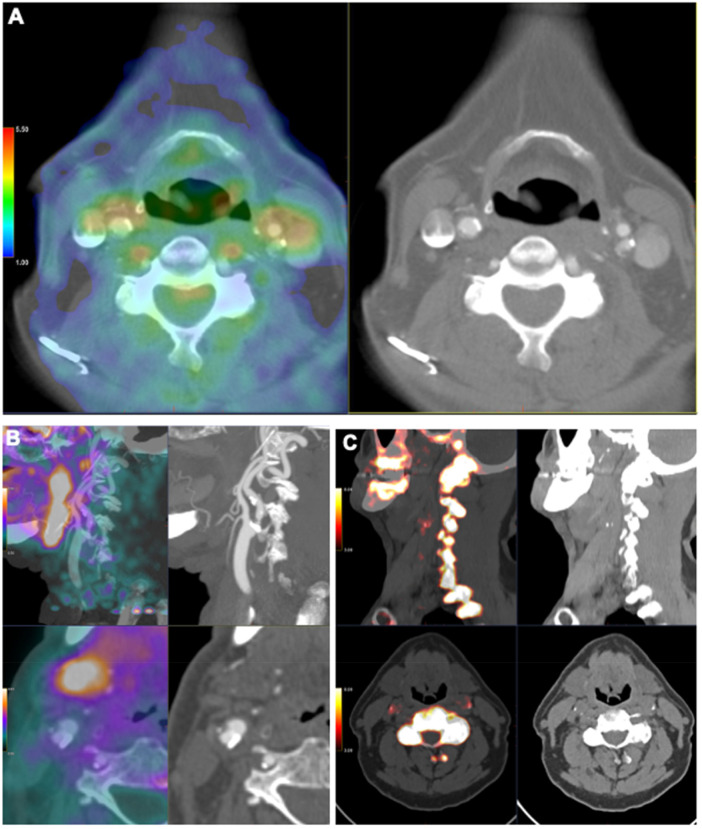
Representative figure demonstrating research examples of PET tracers used to study atherosclerotic plaque. (A): the panel on the left shows used FDG PET/CT angiogram image showing higher activity at the left carotid plaque at the level of proximal internal carotid and on the right the corresponding CT angiogram image showing mixed calcified and non-calcified plaque components; (B): left panels showing fused Ga^68^ DOTATATE PET/CT angiogram image showing higher activity at the right carotid plaque at the level of common carotid bifurcation; right panels showing corresponding CT angiogram image showing mixed calcified and non-calcified plaque components; (C): left panels showing fused NaF PET/CT image showing focal tracer uptake localising specifically to areas of calcification visible on CT and right panels showing corresponding non contrast CT image showing foci of carotid wall calcification.
